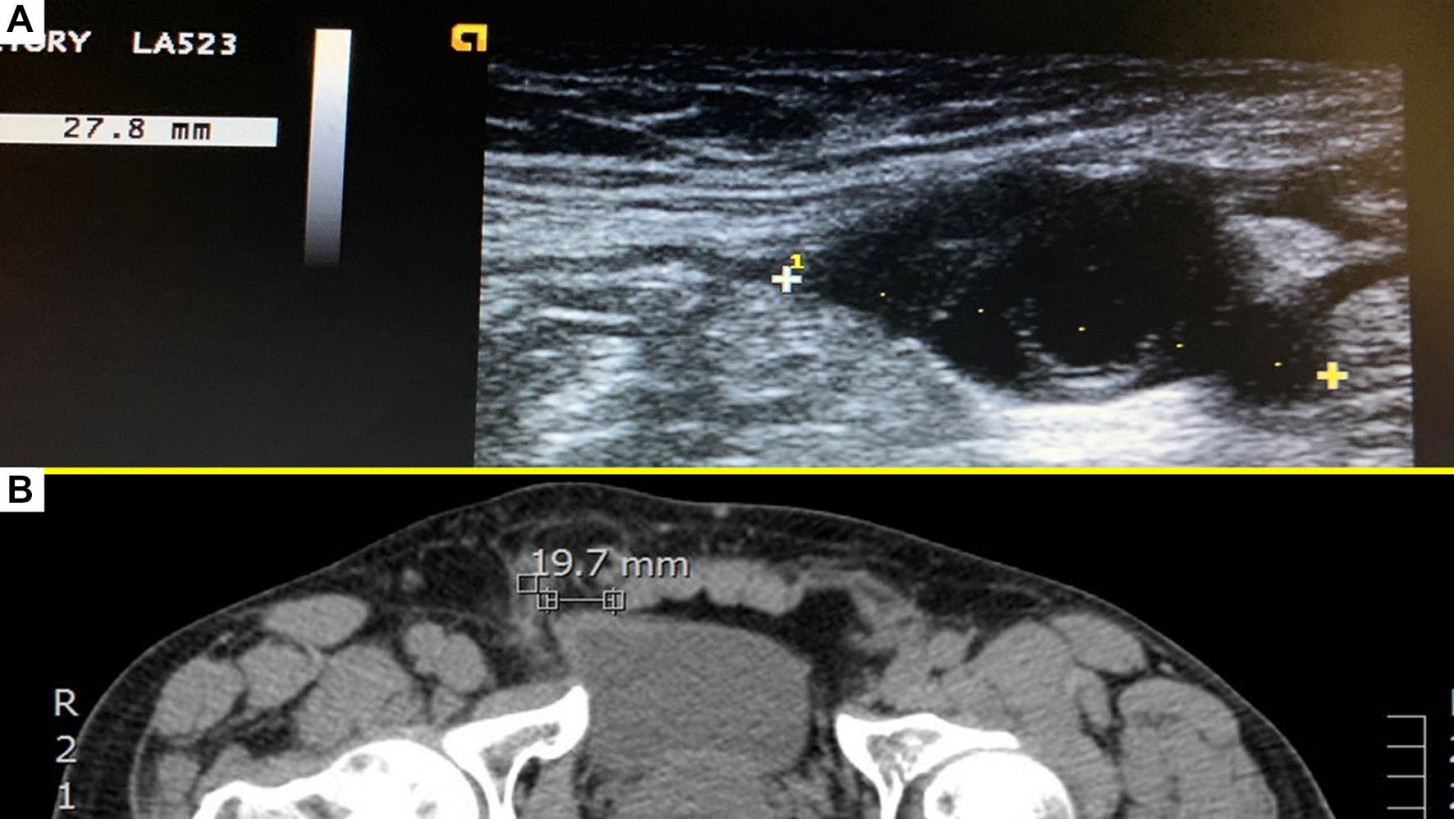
Preoperative hernia defect assessment. (A) Ultrasound image of midsized defect of the inguinal backwall. (B) CT scan showing small inguinal hernia defect in the right groin.
